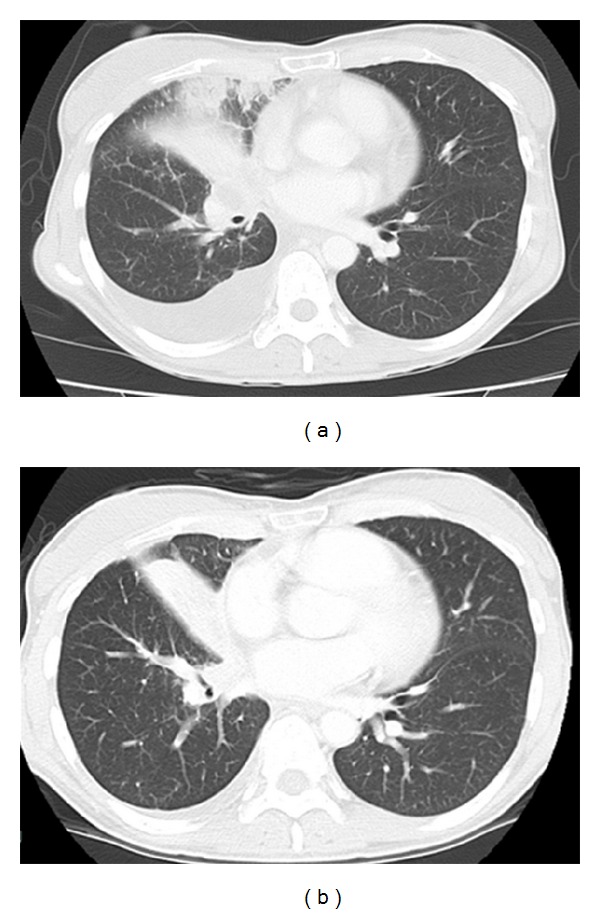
Chest computed tomography (CT) scan before treatment (a) and a month after gefitinib initiation (b). Size reduction of the primary tumor and disappearance of right-sided pleural effusion were observed.
